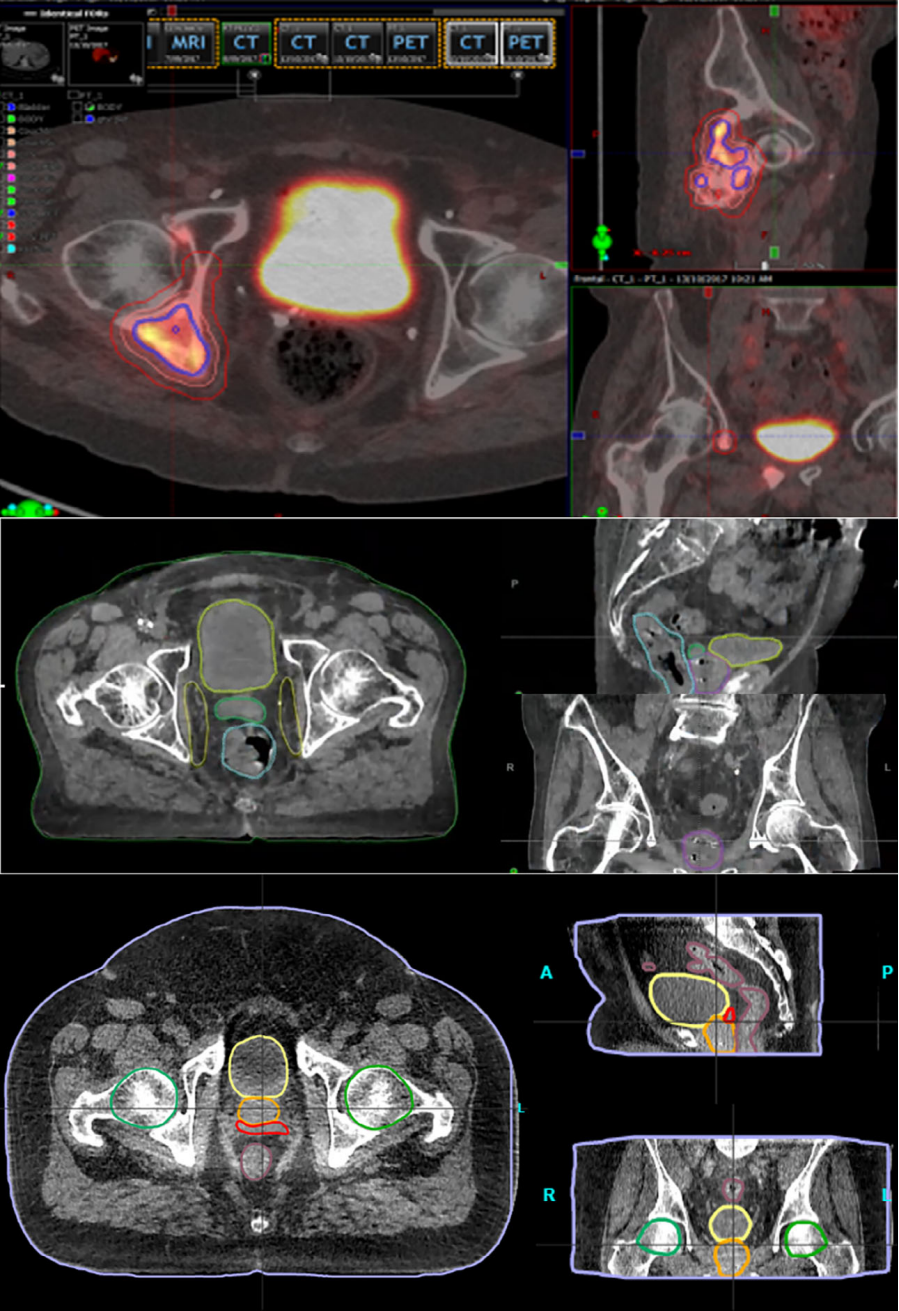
Auto‐segmentation precision examples (A, B, C) on PET‐CT, CT and CBCT guided contouring accuracy for pelvis organs at risk and targets using three current vendor software products.
